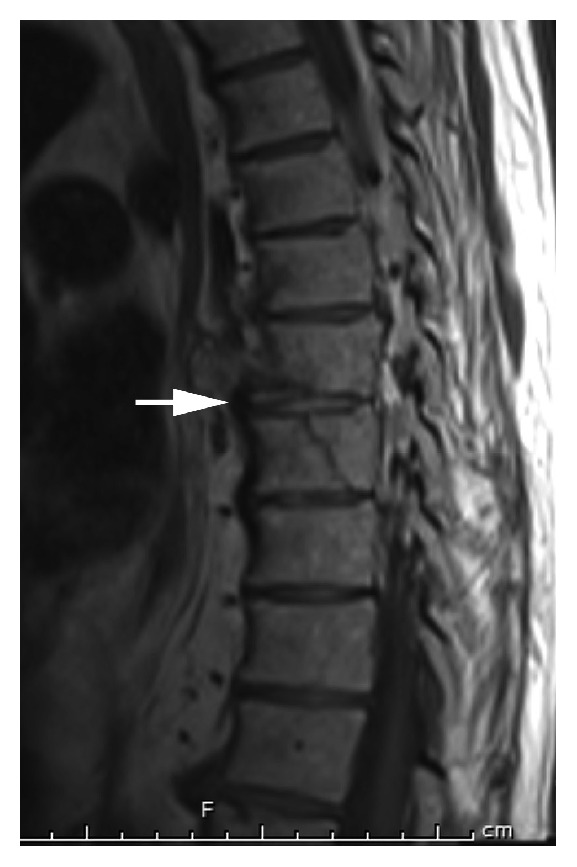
Sagittal MRI, T1 sequence, showing the T8-T9 fractures involving the anterior and middle columns with widening of the anterior disc space (*arrow*).
